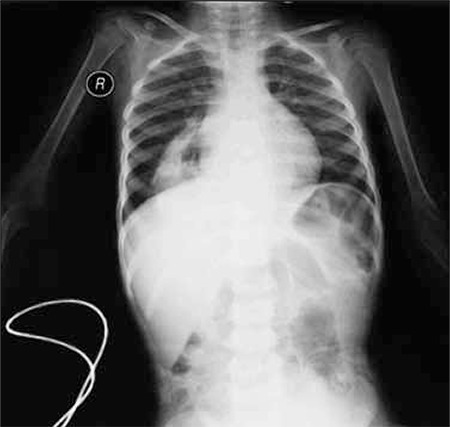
X-ray of the thorax showing the radiolucent area with clear borders in the right paracardiac region.
